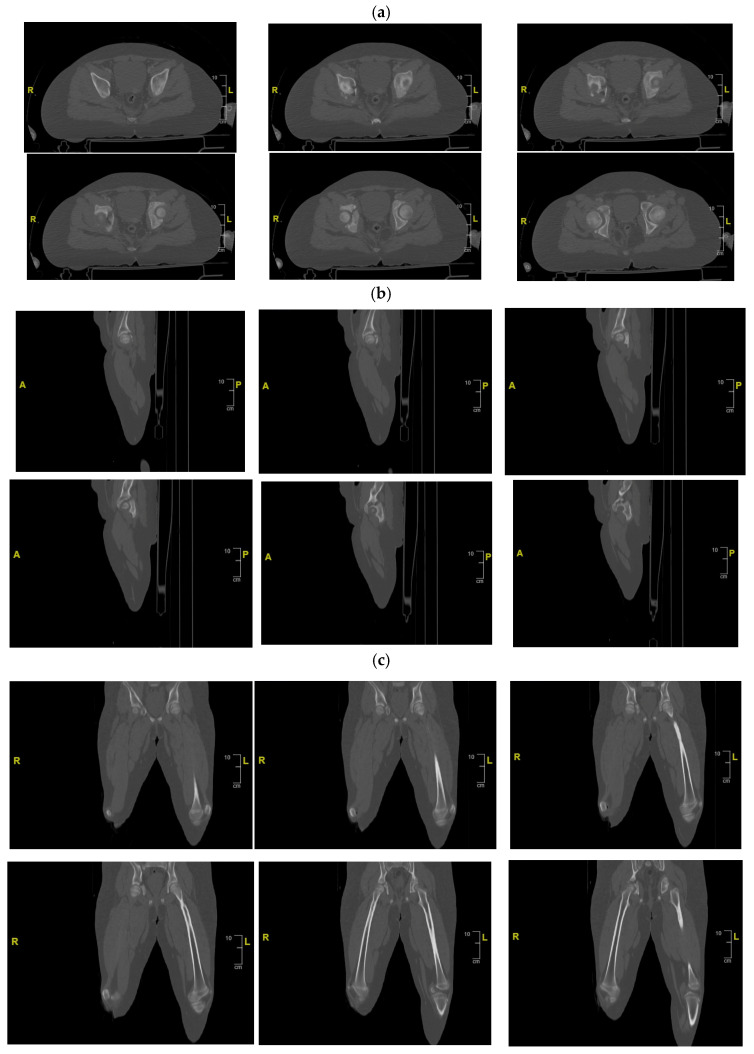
CT of the pelvis showing the right acetabular fracture. (a) Axial view; (b) sagittal view; (c) coronal view. R = right; L = left; A = anterior; P = posterior.
